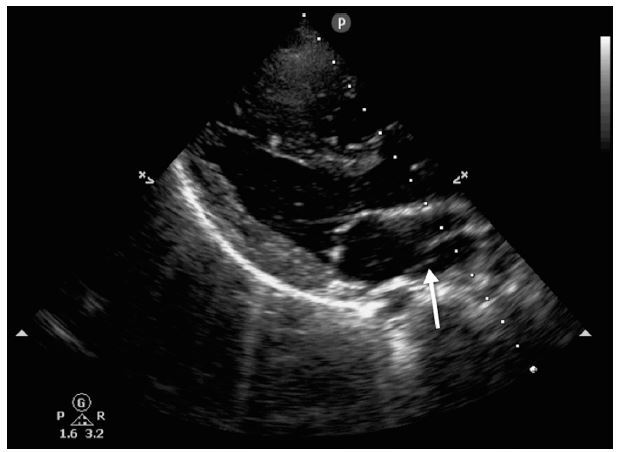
Parasternal long axis view showing double left atrium (arrow).
